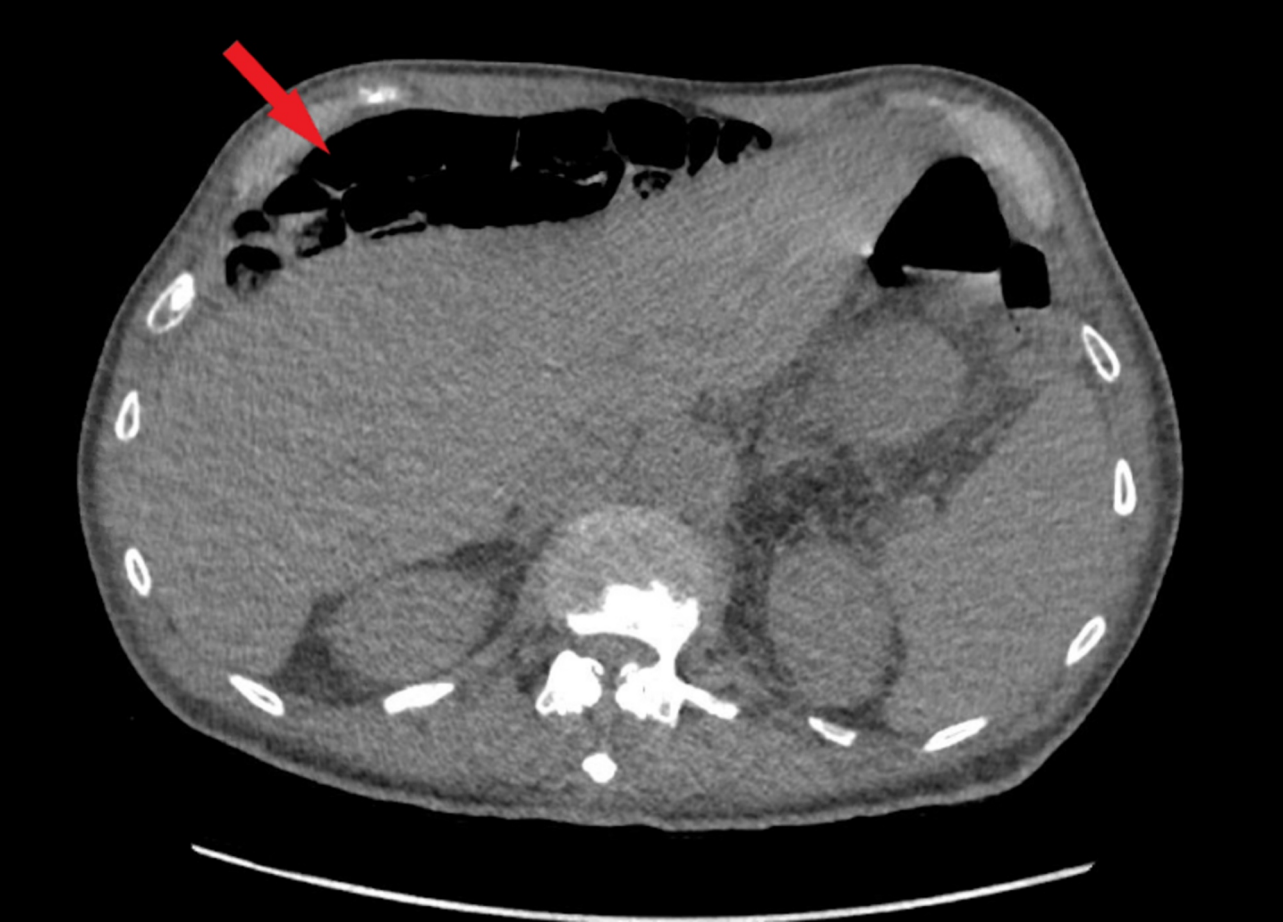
CT of the abdomen. Red arrow shows the interposition of bowel loops anterior to the liver.
